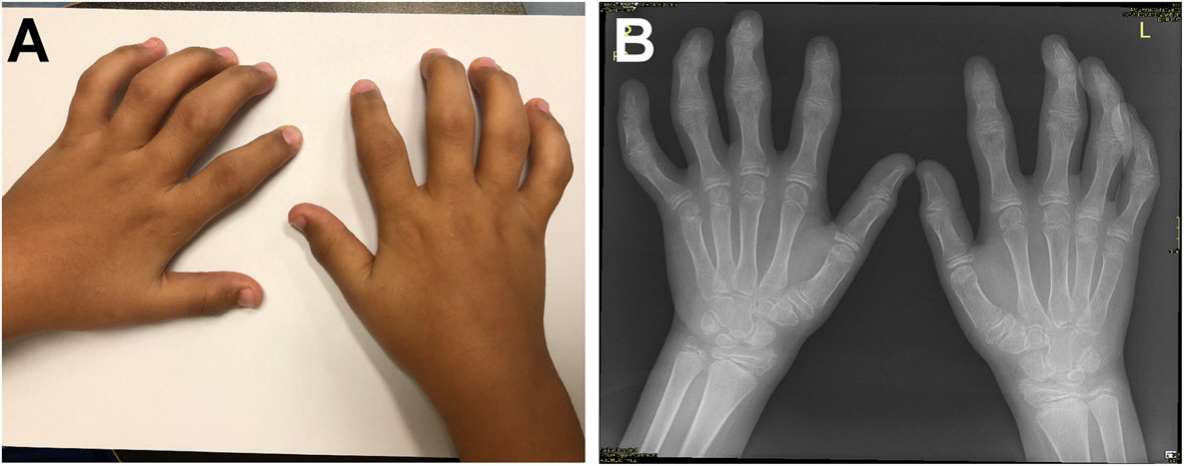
a: Swollen wrists and small joints including the proximal interphalangeal joints (PIPs) and distal interphalangeal joints (DIPs) with bony enlargement, tenderness, and fixed flexion deformities. b: Radiograph shows arthritic changes involving the interphalangeal joints, more in the PIPs associated with enlarged proximal phalangeal epiphyses and metaphyses. The intercarpal joints and wrist joints showed the same osteoarthritic changes
